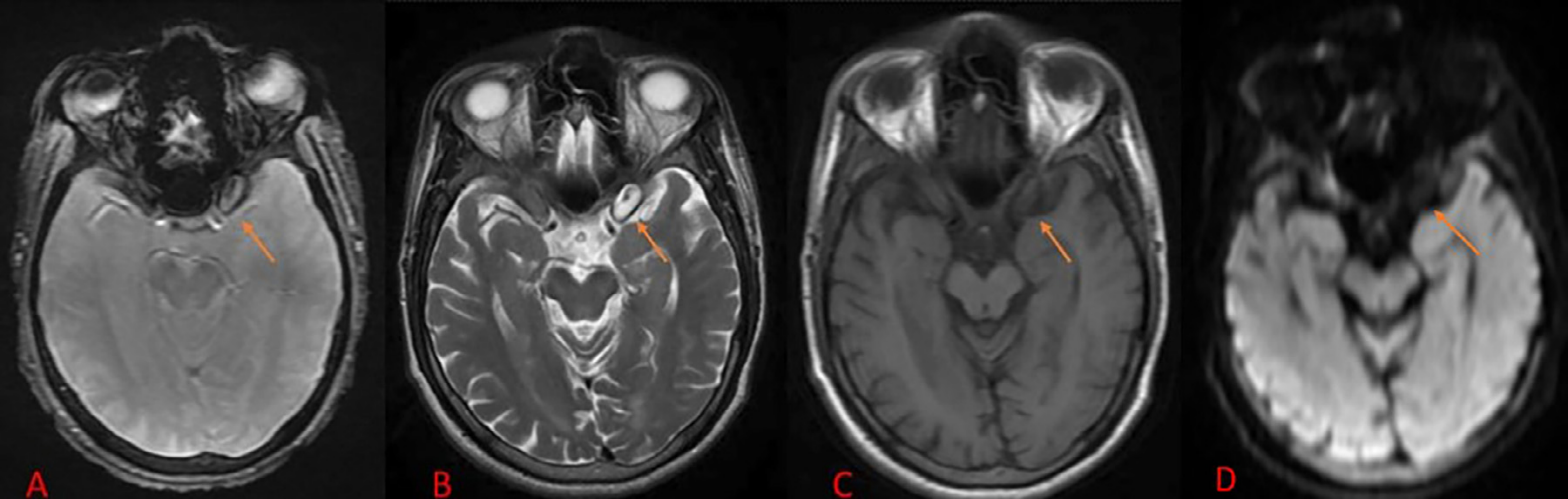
MRI brain axial images of Fluid attenuation inversion recovery sequence (A), T2 weighted imaging (B), T1 weighted imaging (C), and Diffusion Weighted Imaging (D) showing an intra-diploic lesion measuring approximately 14 × 7 mm adjacent to the left cavernous sinus, which appears isointense to CSF on T2WI, hypo to isointense to CSF on T1WI/FLAIR, and no restricted diffusion on DWI.
